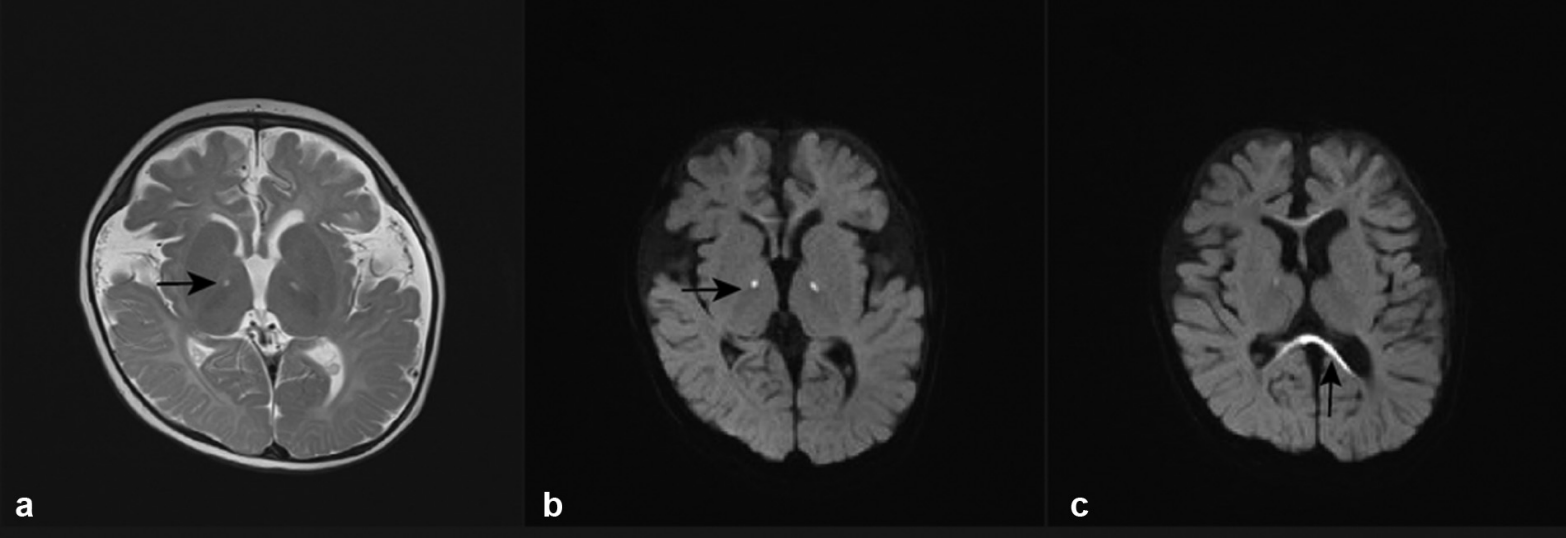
Brain magnetic resonance imaging.
